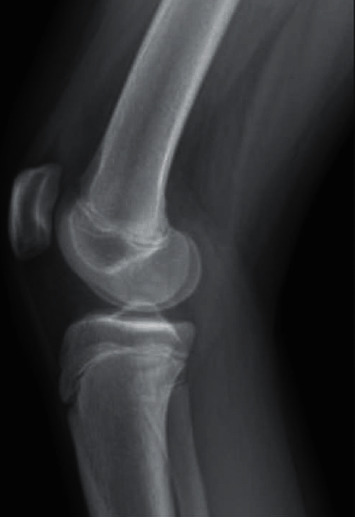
Preoperative lateral view radiograph of the right knee demonstrating open growth plates.
